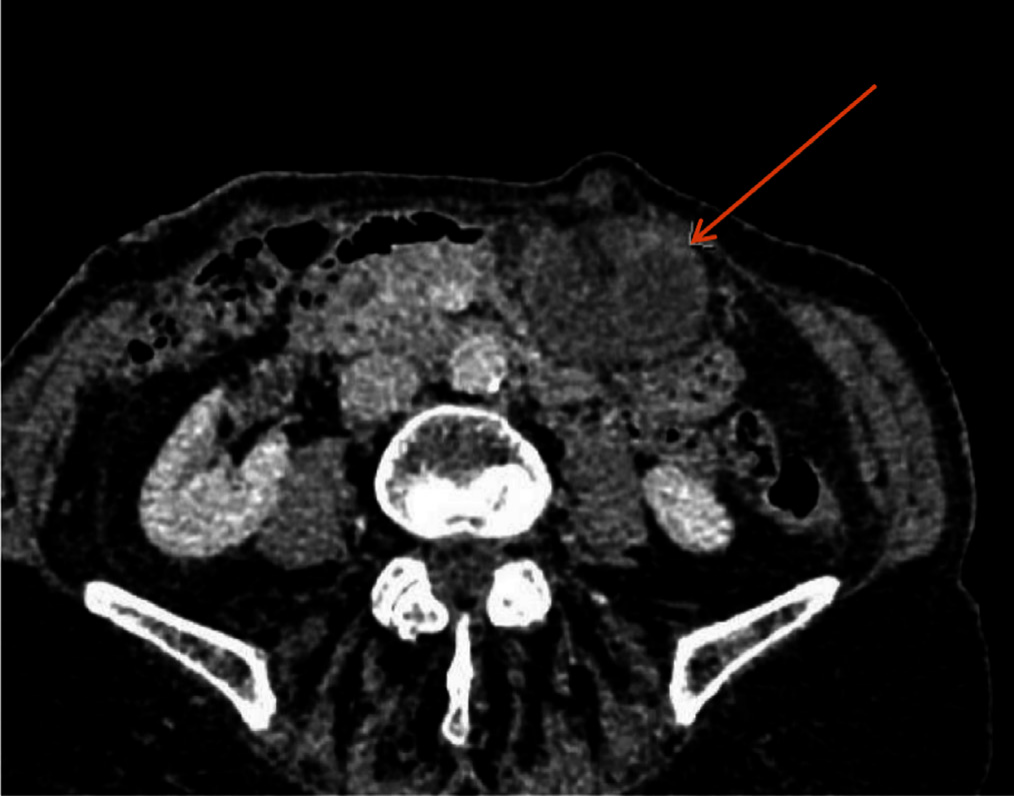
Computed Tomography: Coronal View Coronal view showing the suspected gallbladder with signs of torsion
